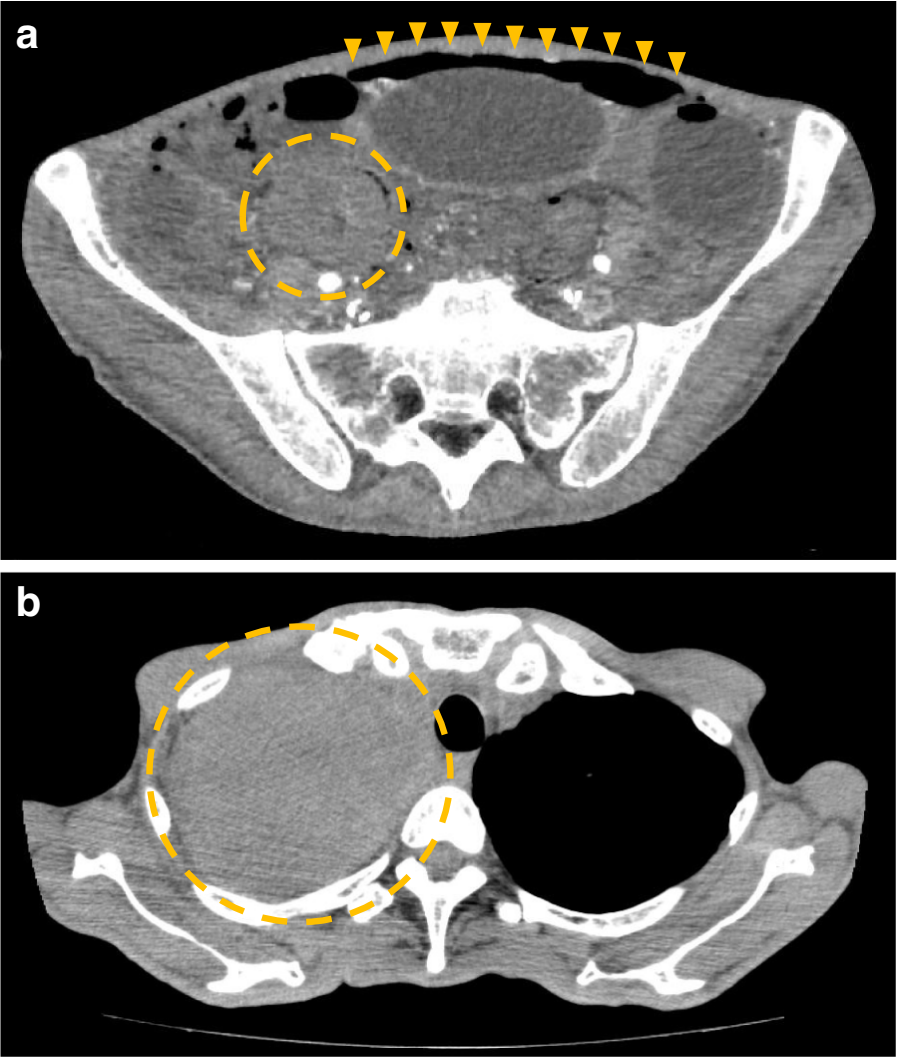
Computed tomography findings. a Intestinal ischemia was noted in almost all intestinal tracts. Extensive free intra-abdominal gas was noted in the upper and lower abdomen (arrowheads). Intestinal pneumatosis and intussusception of the small intestinal tumor were noted in the right lower abdomen (circle). b A large mass was found in the upper right lobe of patient’s lung (circle)
